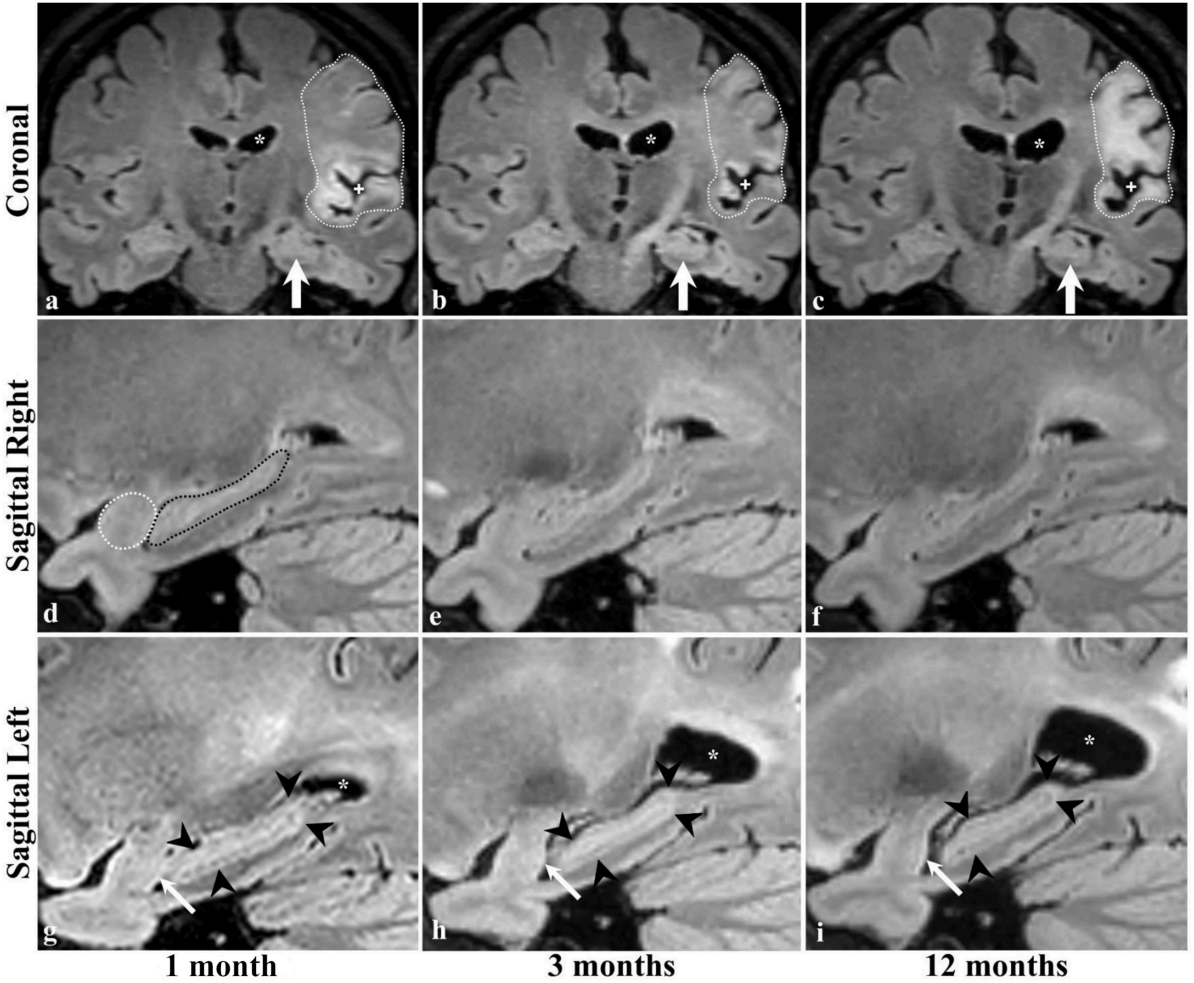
Brain MRI of a patient with ischemic stroke in the left MCA territory. Coronal images are at the level of the interthalamic adhesion at 1, 3 and 12 months of stroke (a–c, respectively), with concurrent sagittal images centered at the right (d–f) and left (g–i) hippocampi. Dotted lines (a–c) delineate the infarcted brain tissue and its expected evolution in time, including the increase in the parenchymal signal intensity (gliosis), the *ex-vivo* dilatation of the corresponding lateral ventricle (^*^ in the top and bottom rows), and the widening of the adjacent subarachnoid space (+ in top row) indicative of parenchymal volume loss and scarring. The left hippocampus was not affected by the ischemic event (arrows in a–c). Dotted lines in the image of the right temporal lobe (d) delineate the normal appearing amygdala (white) and hippocampus (black) and no changes were identified at 3 (e) or 12 (f) months. The left amygdala and hippocampus are normal in size and morphology at baseline examination (g); however, there is progressive hippocampal atrophy in the follow-up scans (arrowheads in g–i). Interval volume loss of the left temporal amygdala can be identified by gradual straightening of its posterior contour (arrows in g–i).
